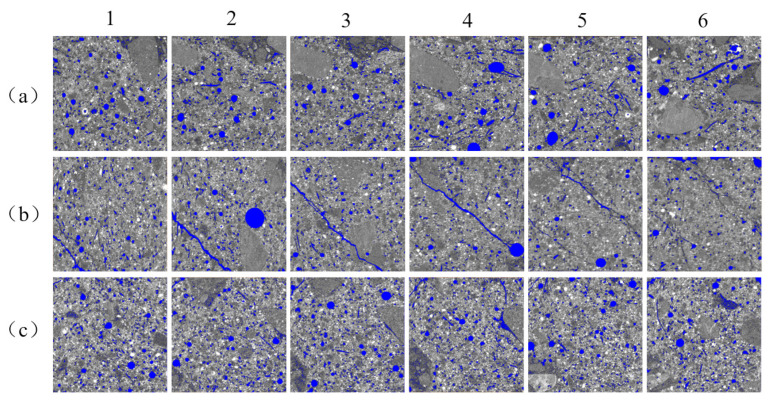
X-CT scanning 2D feature slices: (a) C, (b) S-20, and (c) S-40.
